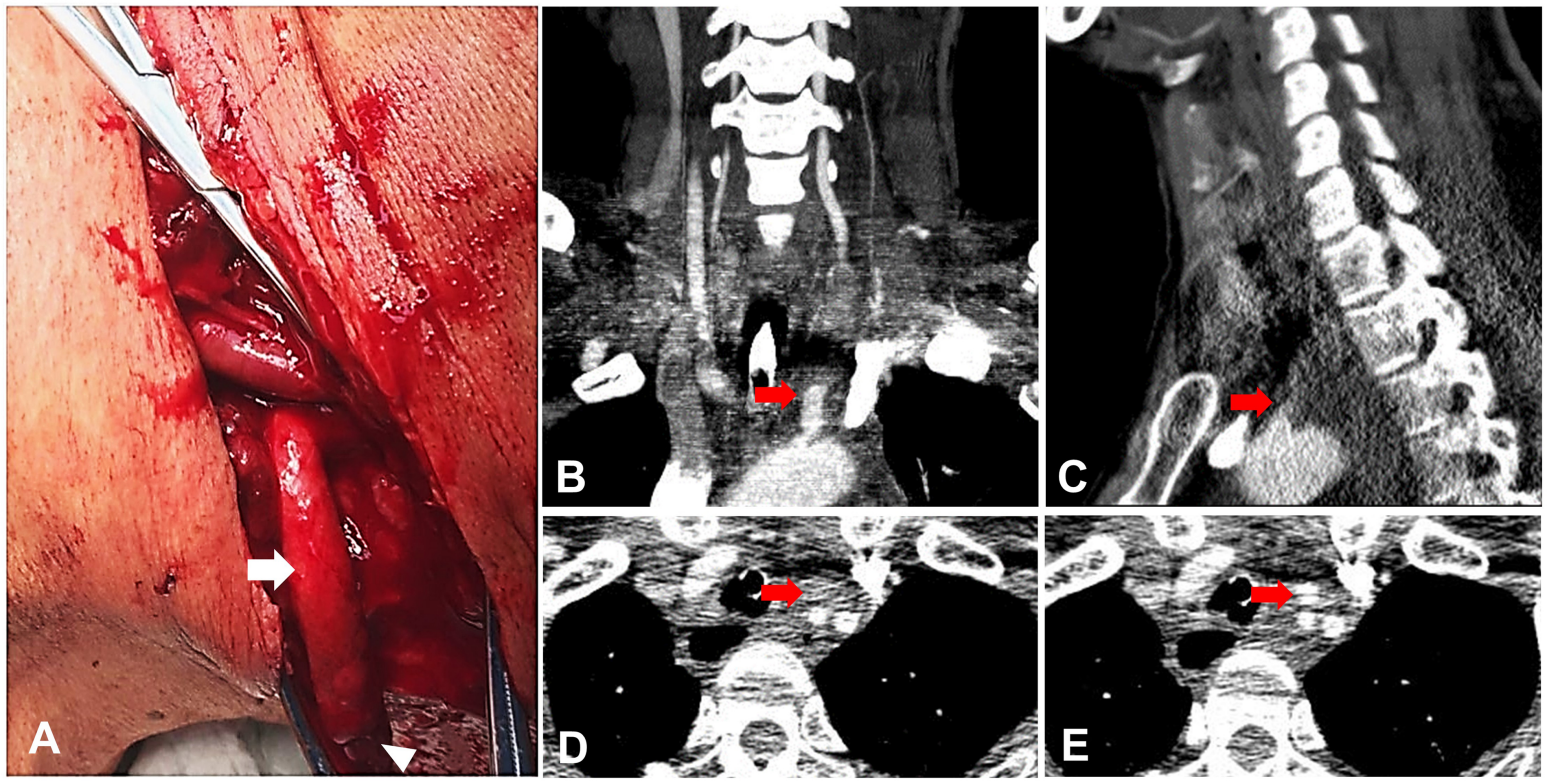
Case 2: (A) the left common carotid artery that was severed from the proximal end of the heart and prolapsed at the time of treatment. Note: the arrow points to the left common carotid artery. The triangle points to the distal end of the left common carotid artery. The cervical CT coronal (B) and sagittal (C) images showed that the origin of the left common carotid artery was not shown, the contrast filling was not seen in the distance, and the structure was not clearly displayed. Note: the arrow refers to the beginning of the left common carotid artery. (D) The left common carotid artery was not seen at the CT level of the neck. Note: the arrow refers to the location of the left common carotid artery. (E) Normal development of the left common carotid artery at the level of the CT in the neck. Note: the arrow refers to the left common carotid artery.
